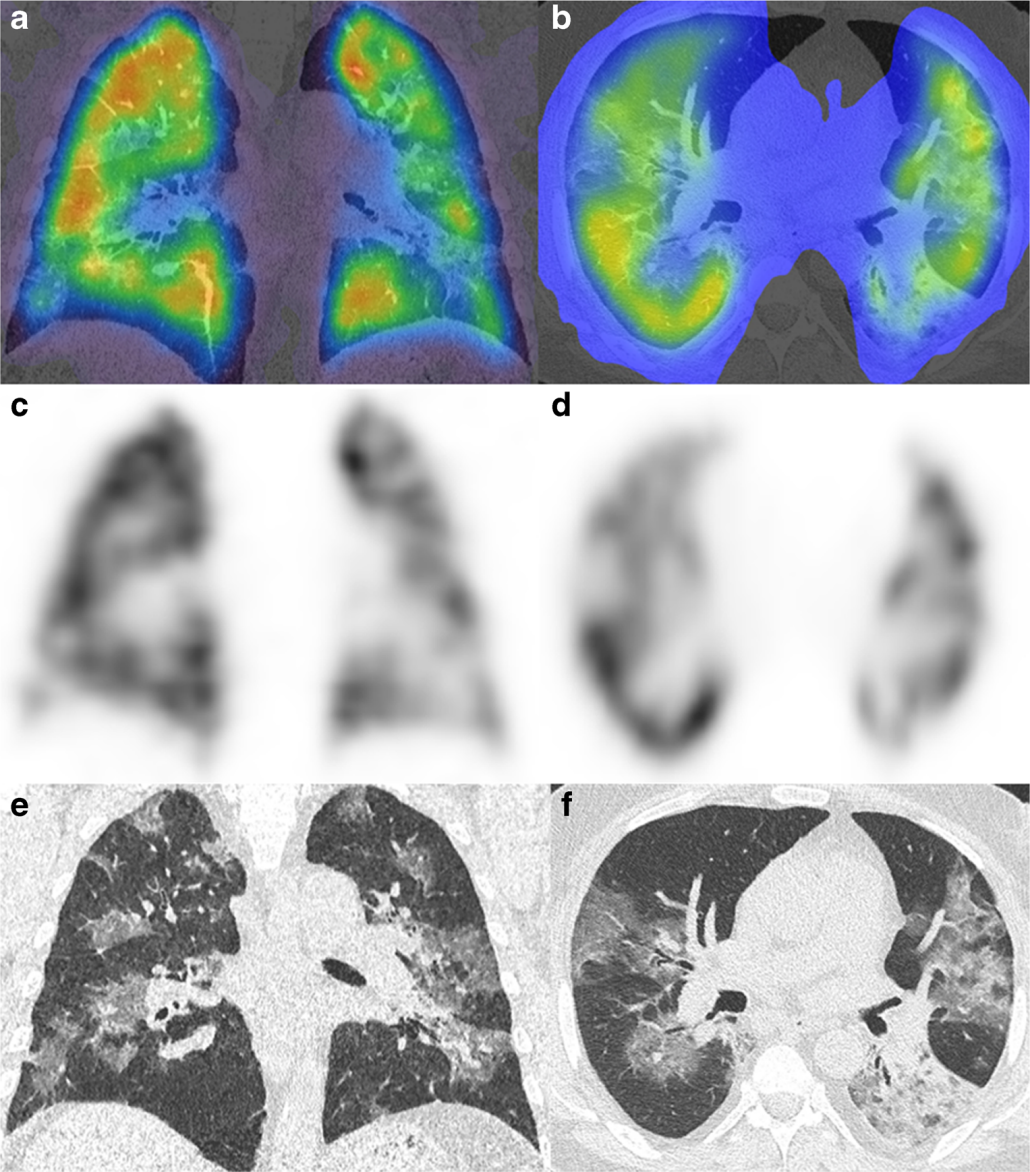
Perfusion SPECT/CT images of a heterogenous perfusion pattern in a case of COVID-19 pneumonia. Shown are the hybrid images (a/b), the perfusion SPECT (c/d), and the non-contrast enhanced CT images (e/f)
